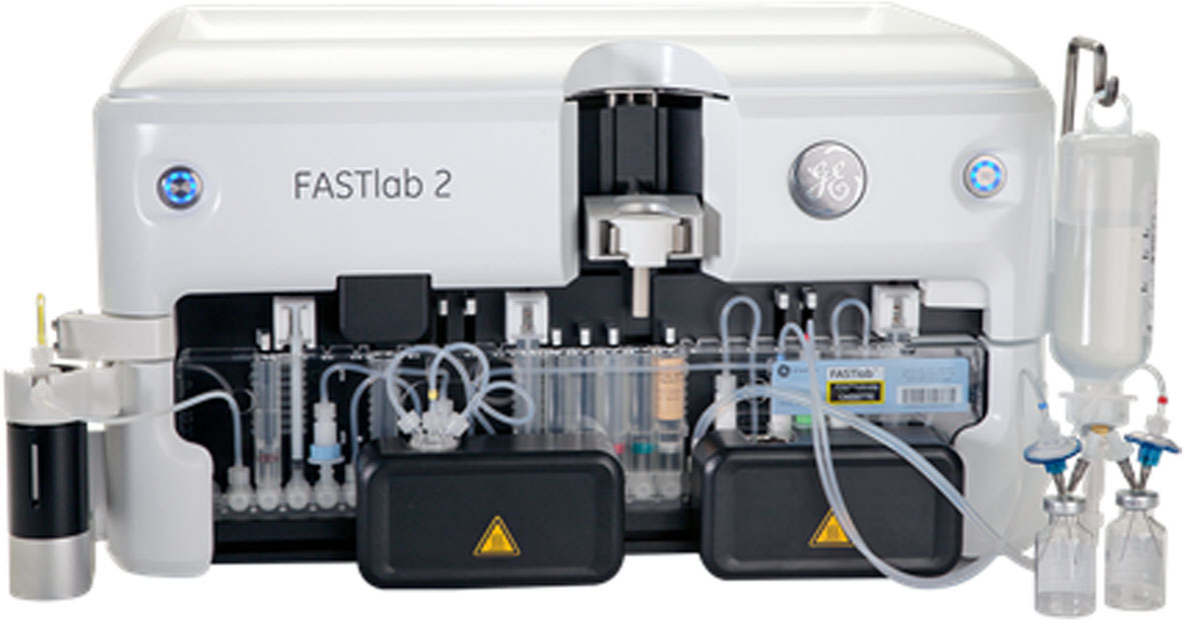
FASTLab 2 equipped with dual run FDG DUO cassette (image courtesy of GE Healthcare)
